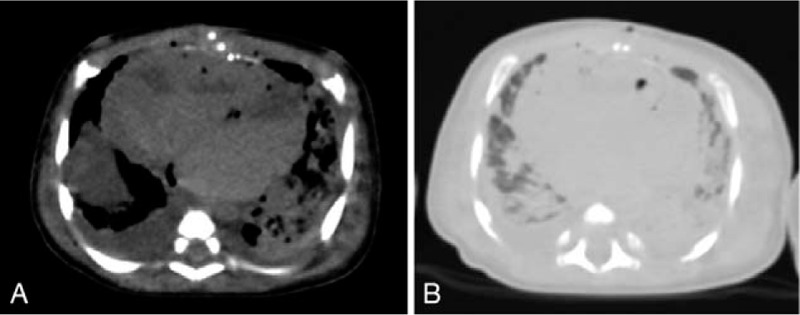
A representative case involving a deceased boy aged 1 month. Computed tomography (CT) was performed 12 h and 11 min after death. (A) A single ventricle and single atrium are suspected on postmortem CT (PMCT). Intracardiac gas due to the resuscitation attempt is also found. (B) Bilateral pulmonary consolidation is observed on PMCT, and the lungs were suspected to be the main organs associated with the immediate cause of death. However, autopsy localized the heart as the main organ associated with the immediate cause of death, which was confirmed to be circulatory failure.
